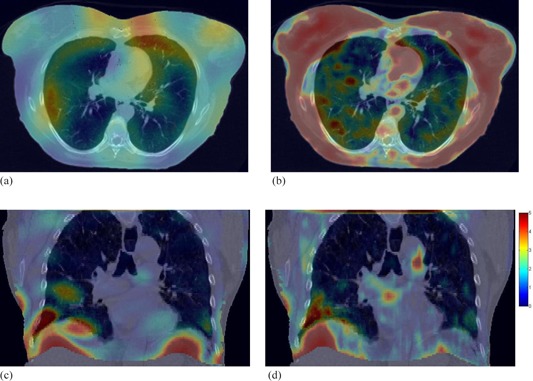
Color overlay of displacement errors with CT image sets. The figure contains an axial cut of image set ${\text{Lung}2}_{2.7\text{Cm}}$ overlaid with the errors of (a) DMP registration and (b) Demons registration. Also shown is a coronal slice of ${\text{Lung}1}_{3\text{Cm}}$ overlaid with (c) DMP and (d) Demons registration errors. Error values seen in the color bar (d) are given in mm.
